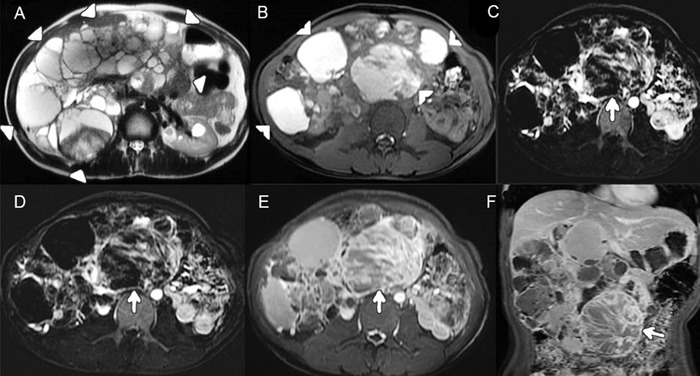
(A) Axial T2-weighted image shows markedly enlarged right kidney (outlined by triangles) studded with multiple cysts, a few containing heterogeneous contents. Some cystic lesions are also seen in the normal sized left kidney. (B) In this axial T1-weighted image, some cysts show T1 hyperintensity suggestive of hemorrhagic/high mucin contents (arrowheads). (C) Corticomedullary phase and (D) nephrographic phase postcontrast (subtracted) axial T1-weighted images reveal progressive enhancement in one of the cysts (arrows) with multiple enhancing septations. (E) Axial 3-minute and (F) coronal 5-minute delayed postcontrast (nonsubtracted) T1-weighted images show further progressive enhancement of the wall of the cystic lesion and septations.
